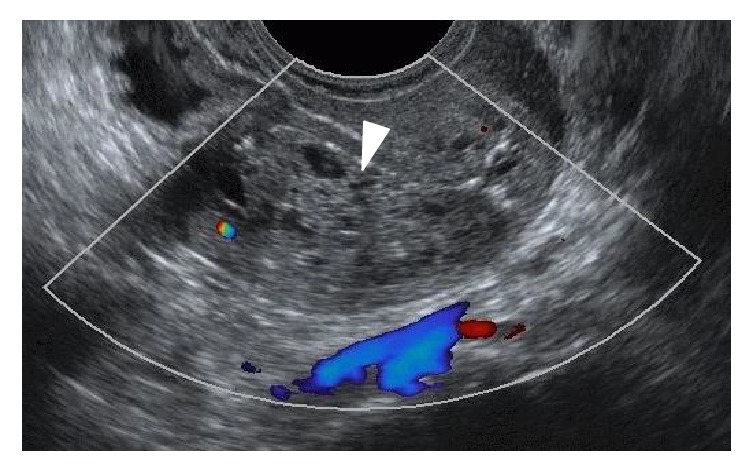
Figures on transvaginal color Doppler ultrasonography, showing a mass in the uterine cavity, measuring 3 × 2 cm and consisting of multiple cysts differing in size (triangle) and without pulsatile blood flow.
